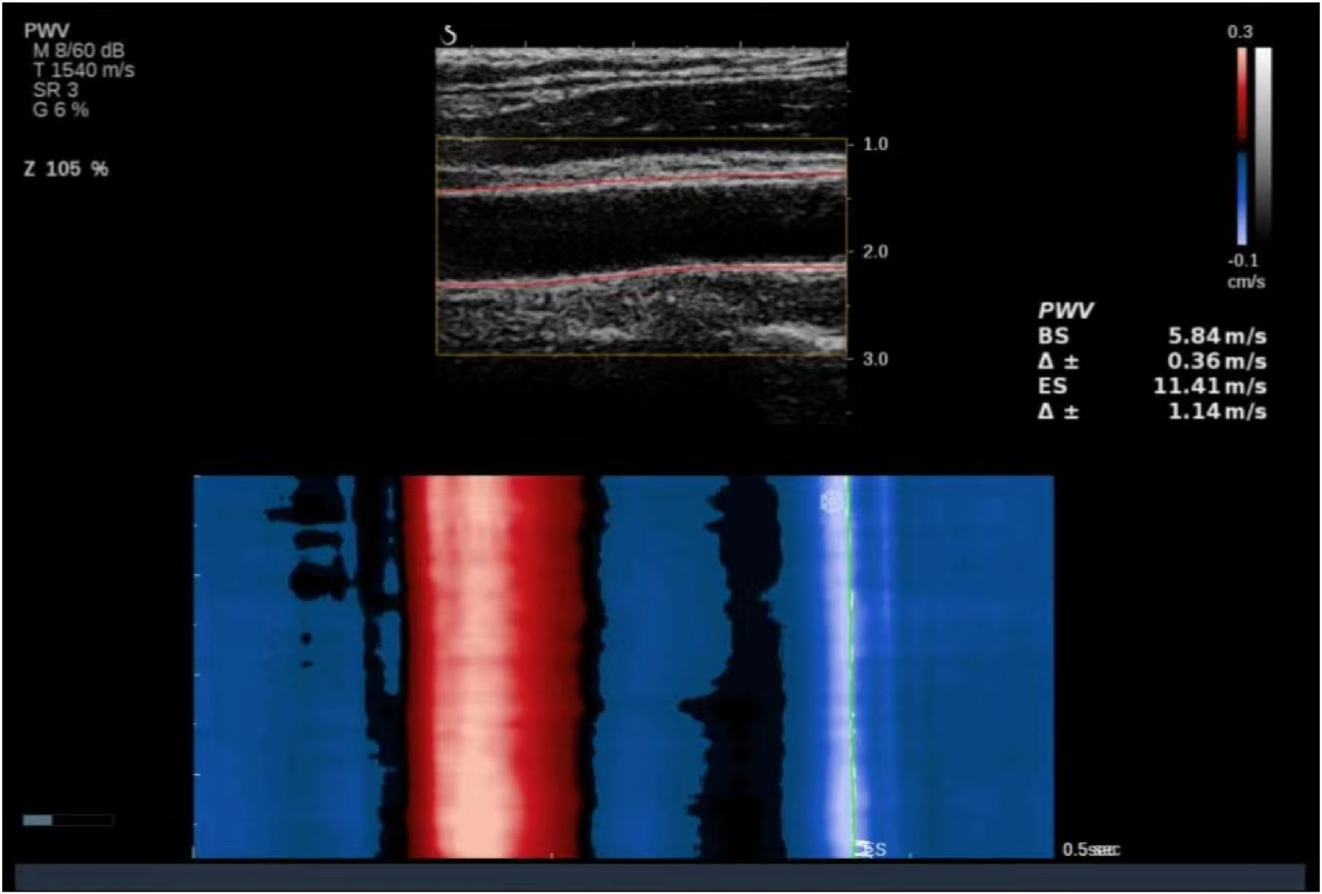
Representative images of PWV measurements at ES and BS using ultrafast ultrasound imaging. The yellow rectangular box represents the area of interest. The two red lines represent the anterior and posterior walls of the auto-tracked common carotid artery. The lumen between the two red lines is the carotid artery. The software can automatically identify and record PWV at BS and ES. The Δ ± values indicated the variance in PWV measurements and should be kept below 20% of the PWV values
